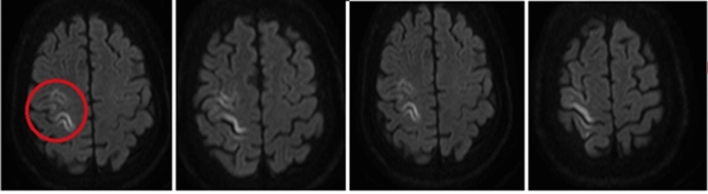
Ischemic stroke: nuclear magnetic resonance image (MRI) showed hyperintensity in this location evaluated in FLAIR: red circle
